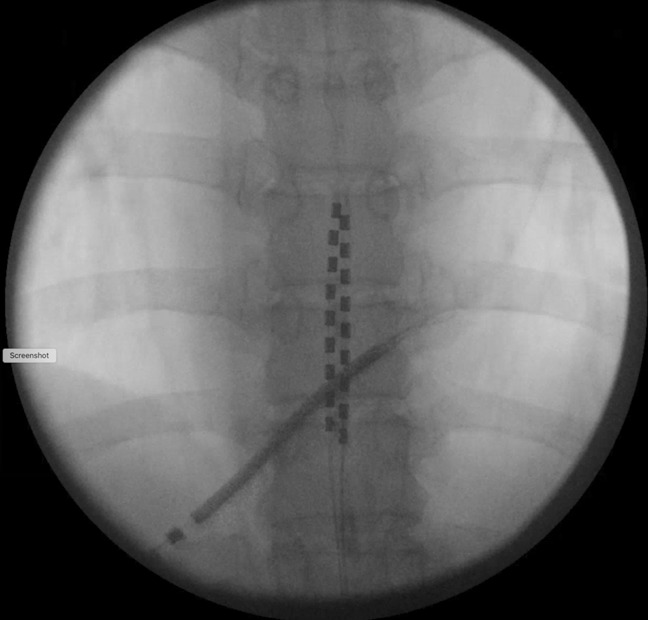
Intraoperative radiograph of the spinal cord stimulation device implantation.
